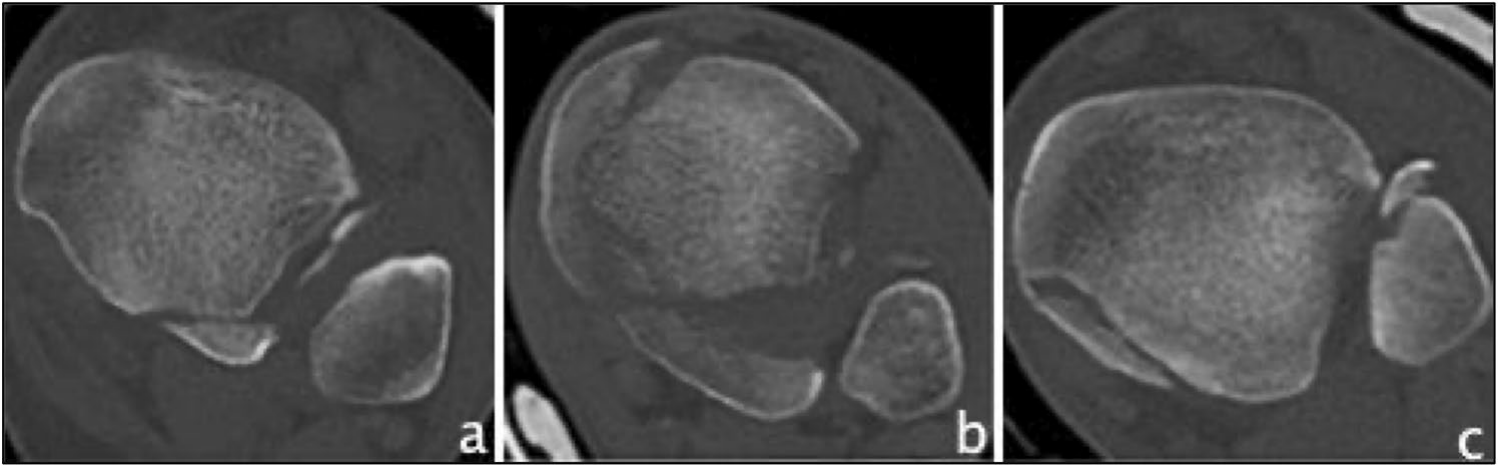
Overview of the Haraguchi classification based on CT images (axial views). a Haraguchi type I b Haraguchi type II c Haraguchi type III
